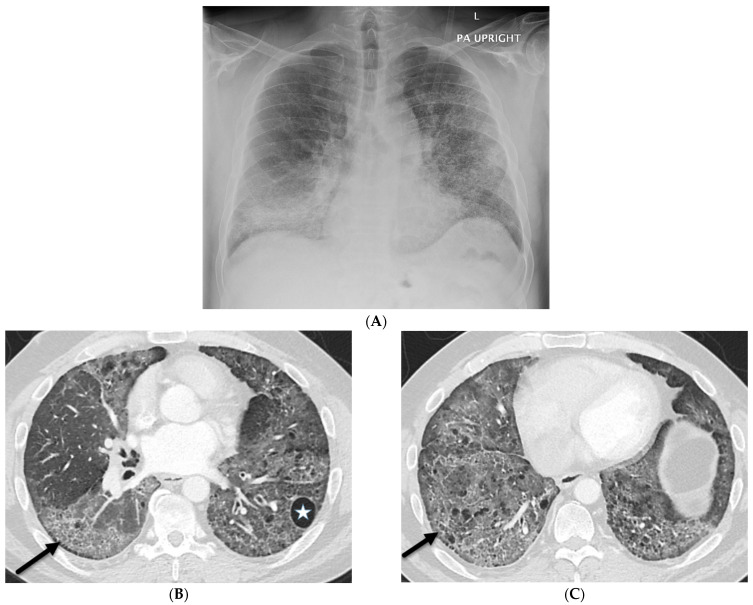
A 47-year-old male with a history of scleroderma and positive ANA titers presents with a cough. A frontal chest radiograph (A) demonstrates interstitial prominence in both lungs, the left greater than the right. Axial chest CT images (B,C) demonstrate widespread basilar predominant ground glass opacities, interlobular septal thickening (solid black arrows), and numerous cysts (star).
